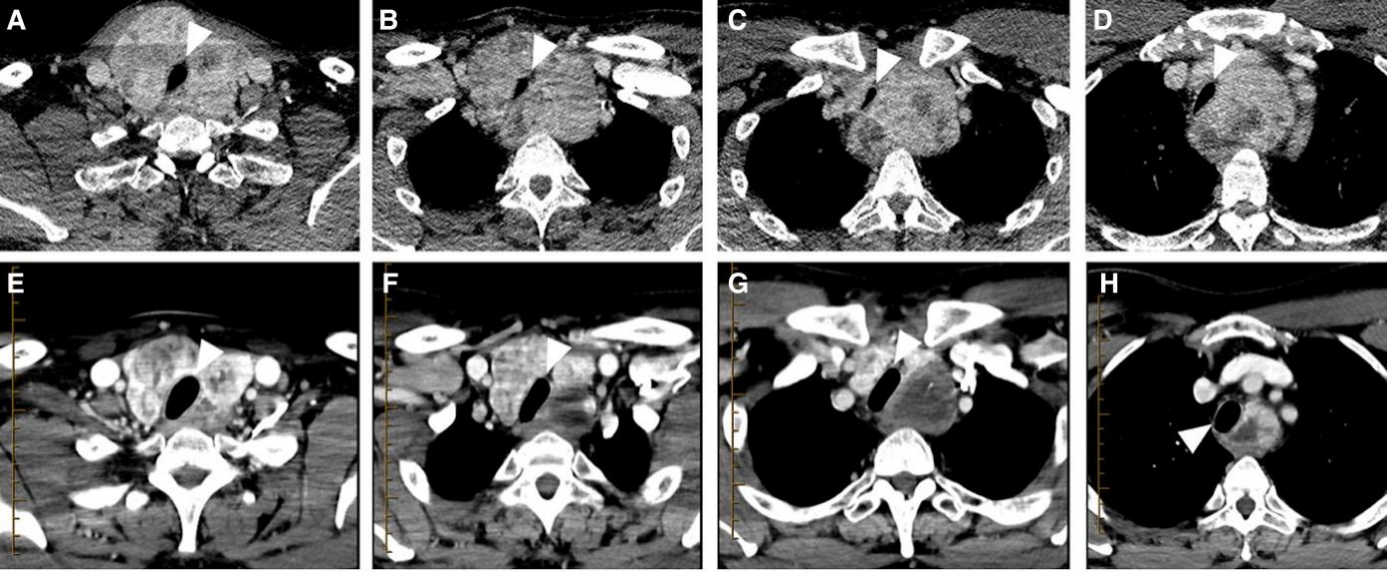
Multinodular goiter case. Preprocedural computed tomography (CT) of the neck images with contrast through the A, infraglotic supraclavicular region; B, at the level of the clavicle; C, below the clavicle; and D, in the superior mediastinum 2 cm above the carina, demonstrating a significant multinodular goiter with mediastinal extension and extreme luminal narrowing (>90%) in a 50-year-old patient presenting with dyspnea on exertion and stridor. Corresponding 3-month postprocedural CT neck images in the D, infraglotic supraclavicular region; E, at the level of the clavicle; F, below the clavicle; and G, in the superior mediastinum 2 cm above the carina demonstrate significant goiter volume reduction with reexpansion of the trachea.
